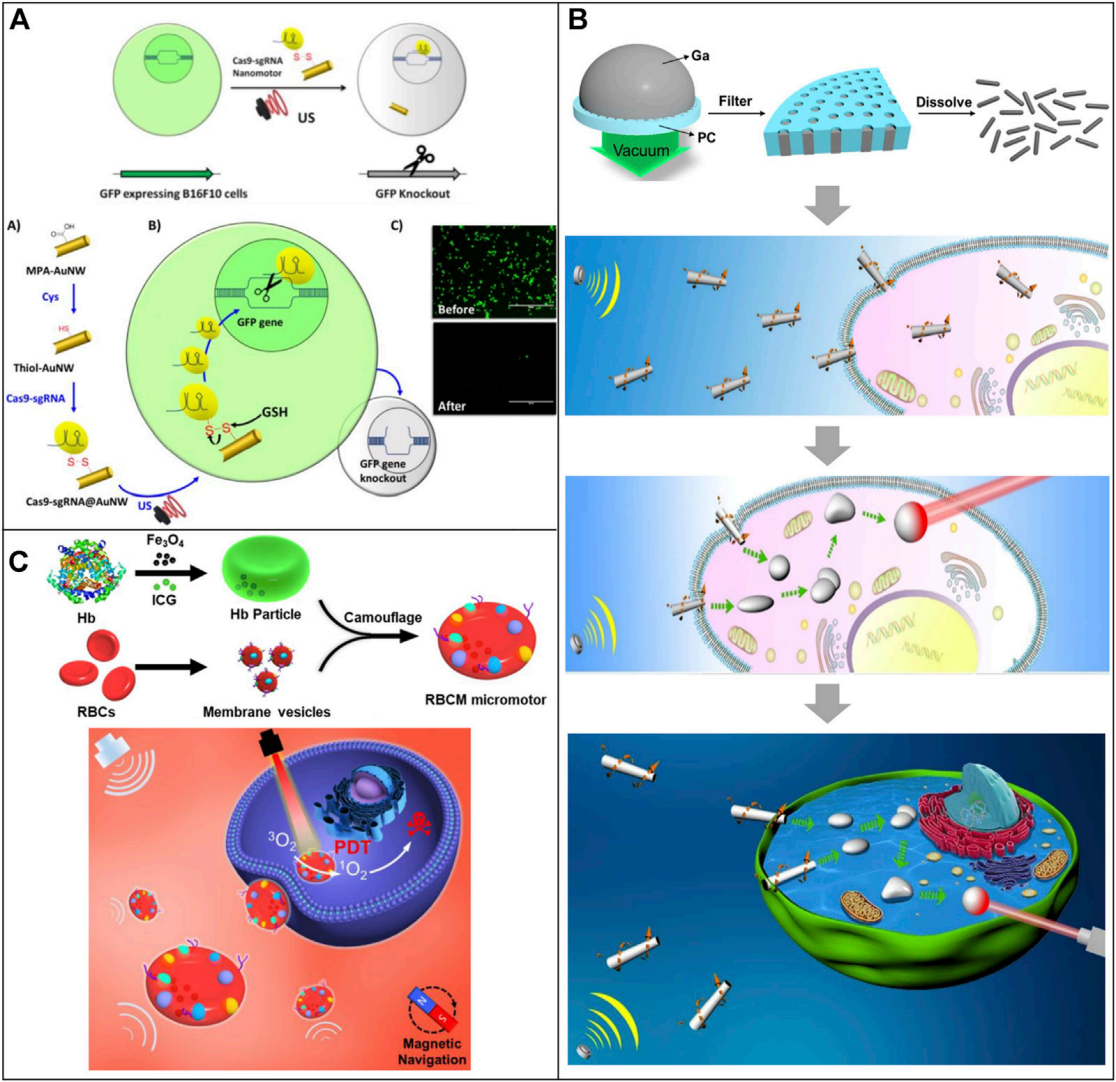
Motile drug delivery by ultrasound-powered MNRs. (A) Red blood cell mimicking-micromotor. (Hansen-Bruhn et al., 2018) Copyright 2018. Reproduced with permission from John Wiley and Sons. (B) Shape-transformable, fusible rodlike swimming liquid metal nanomachine. (Wang et al., 2018) Copyright 2018. Reproduced with permission from American Chemical Society. (C) Cas9-sgRNA@gold nanowire (AuNW) motor. (Gao et al., 2019) Copyright 2019. Reproduced with permission from American Chemical Society.
